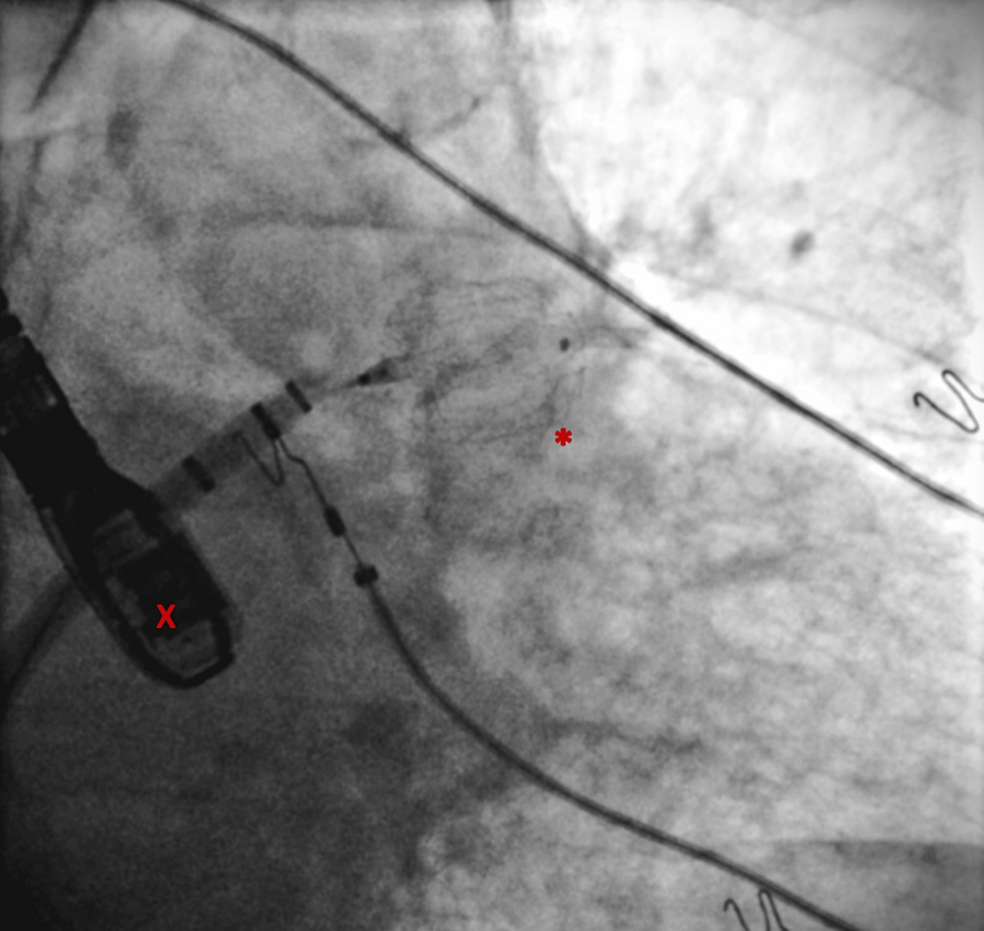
27 mm Watchman FLX being deployed after transseptal access, with TEE and X-ray fluoroscopy guidance. * = Deployed Watchman FLX; X = TEE (transesophageal echocardiogram)
